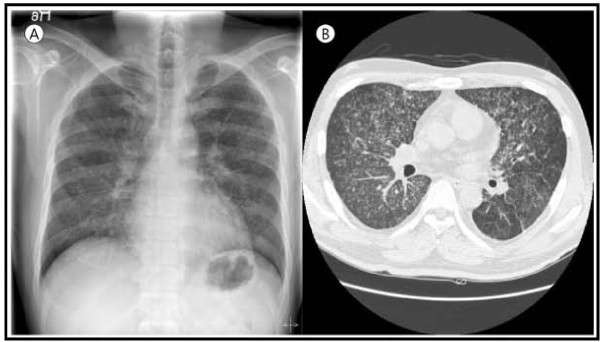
(A) In chest radiographs, the miliary nodules were observed in both lung field. ( B) Chest CT scan showed miliary nodulation and patch underlying perinodular ground glass appearance in both lung field, suggestive of miliary TB.
